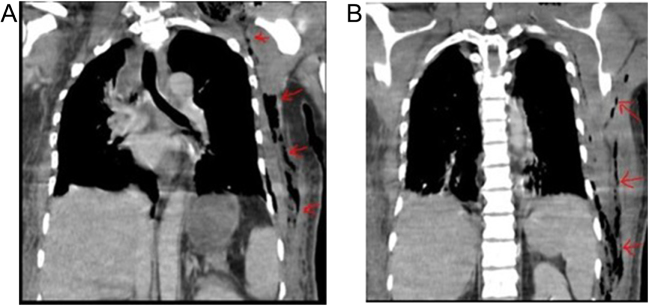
(A and B) Coronal chest CT image shows the lateral chest wall and suprascapular extension of the subcutaneous emphysema.
